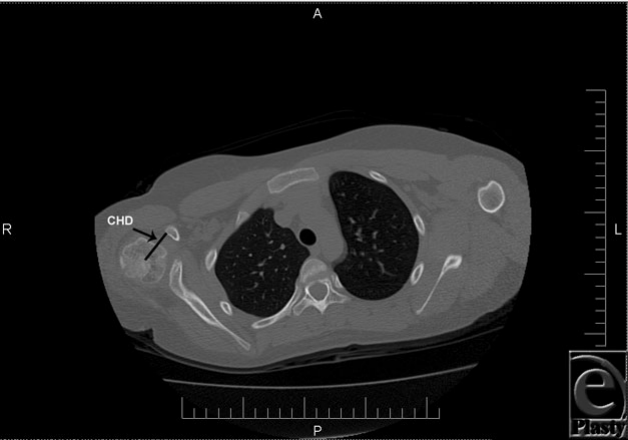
Preoperative computed tomography of a patient with obstetric brachial plexus injuries included in this study showing coracohumeral distance, labeled “CHD.” Scale is shown at bottom. Space between each hache mark is 10 mm. CHD = 35 mm.
